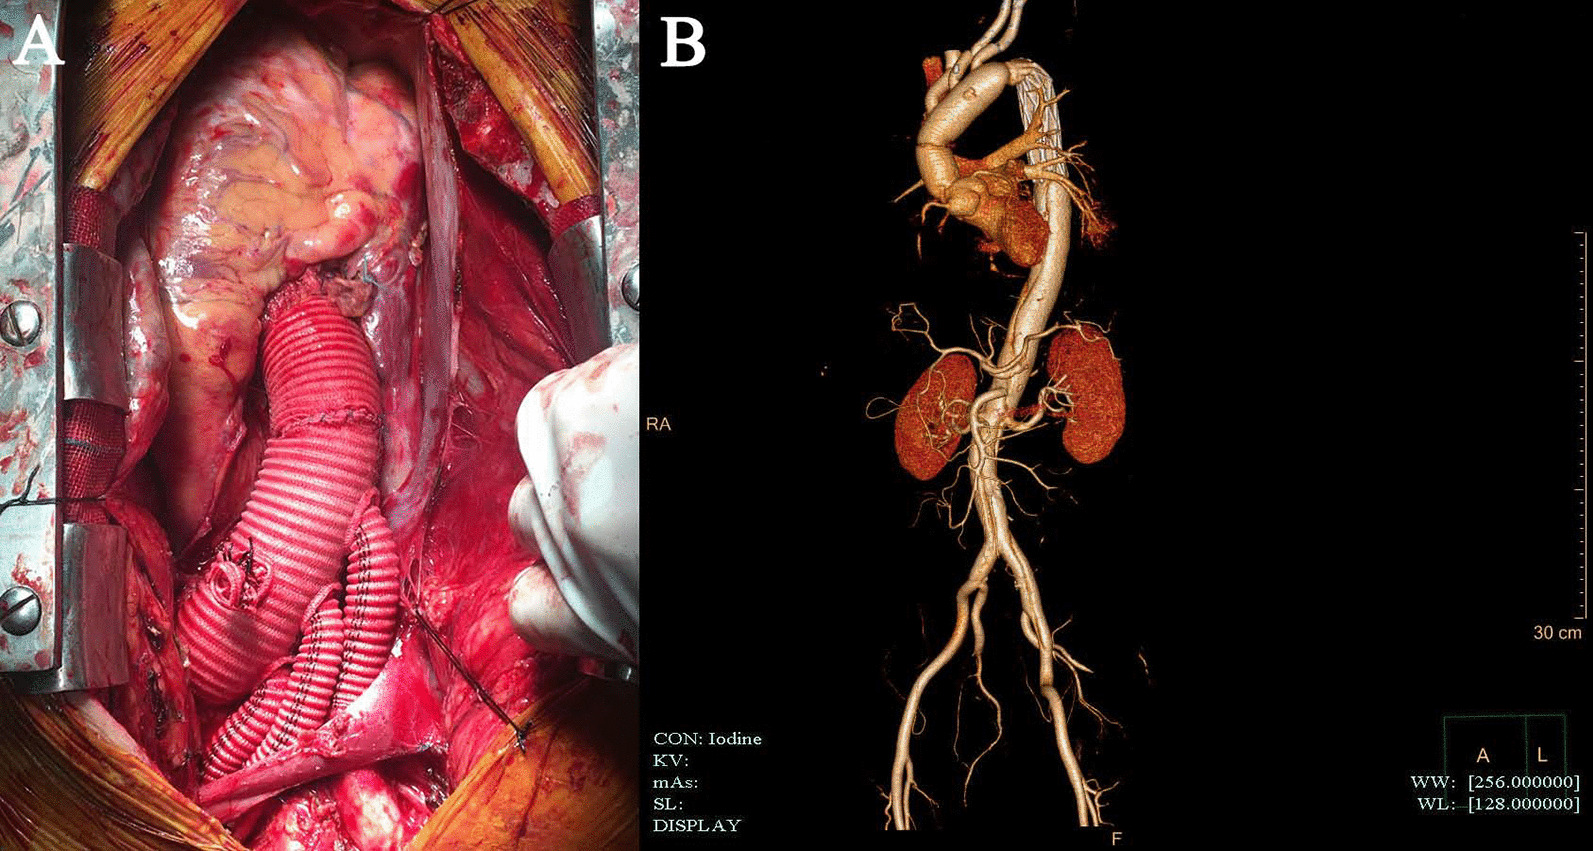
a Final aspect of the surgery. b Postoperative 3-dimensional computed tomography of the aorta
